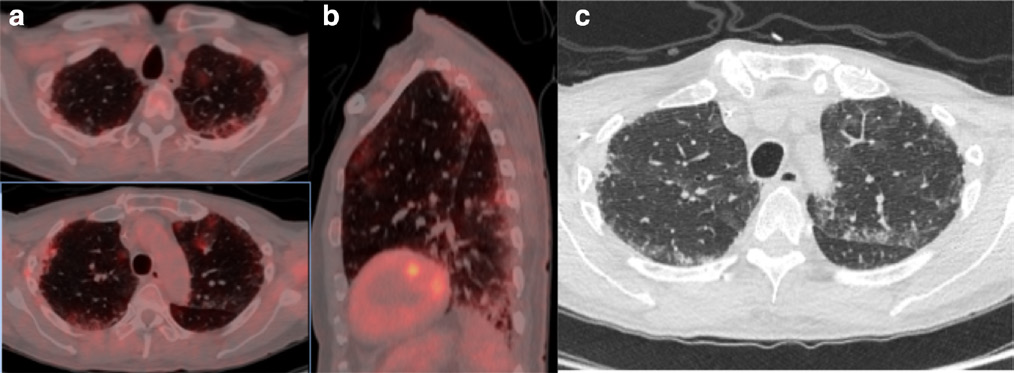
(a) Axial fused thoracic ^18^FDG-PET/CT, (b) sagittal fused thoracic ^18^FDG-PET/CT, (c) axial low-dose CT thorax. The images demonstratemultifocal biapical mildly avid GGO. ^18^FDG-PET, 18-fludeoxyglucose-positron emmision tomography; GGO, ground glass opacity.
